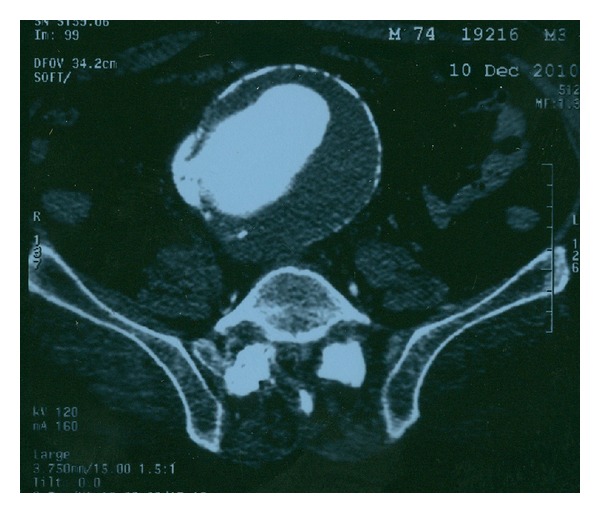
Abdominal CTA revealing a large 9.8 cm AAA with communication between the aorta and the IVC, without evidence of retroperitoneal rupture.
